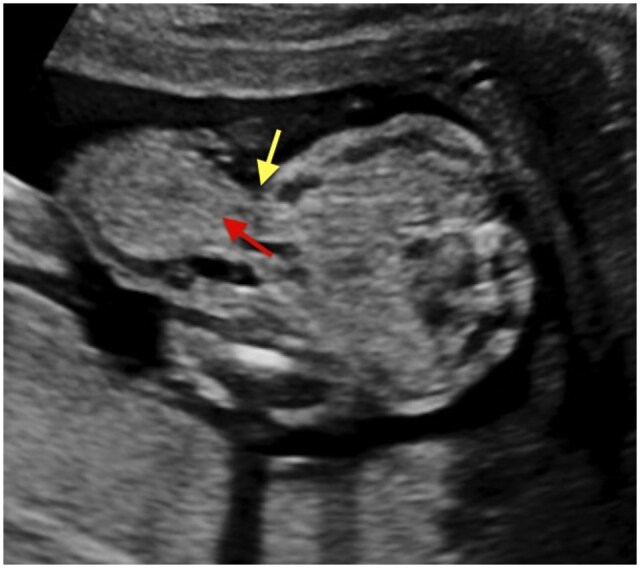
A mid-trimester obstetric ultrasound, shows a small midline anterior abdominal wall defect (red arrow) at the umbilical ring with herniation of bowel loops into the umbilical cord. The herniated contents are covered by intact skin, and no liver tissue is present—features consistent with a congenital hernia of the umbilical cord. A normally inserted umbilical cord (yellow arrow) distinct from the bowel-containing sac, helping differentiate this condition from omphalocele, where the cord inserts at the apex of the sac is visible.
